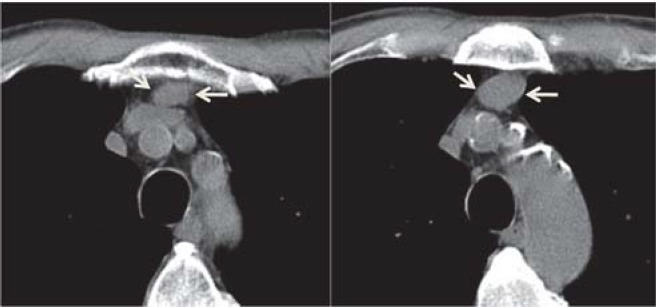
66-y/o male with myasthenia gravis; CT scan shows homogeneous anterior mediastinal mass with round-ovalar shape and smooth contours (arrows); pathologic diagnosis - type A thymoma
